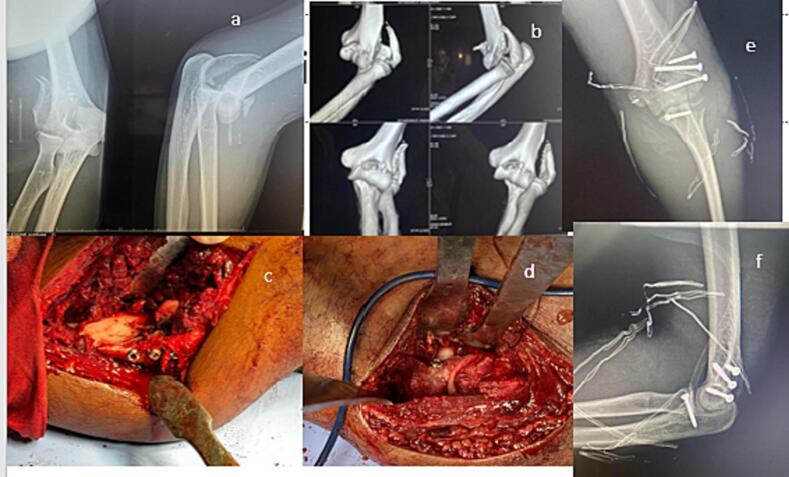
a. Radiological assessment on admission b. 3D reconstruction of the dislocated elbow fracture c. External approach with osteosynthesis of the lateral epicondyle d. Internal approach with osteosynthesis of the coronoid e, f Control X-ray.
